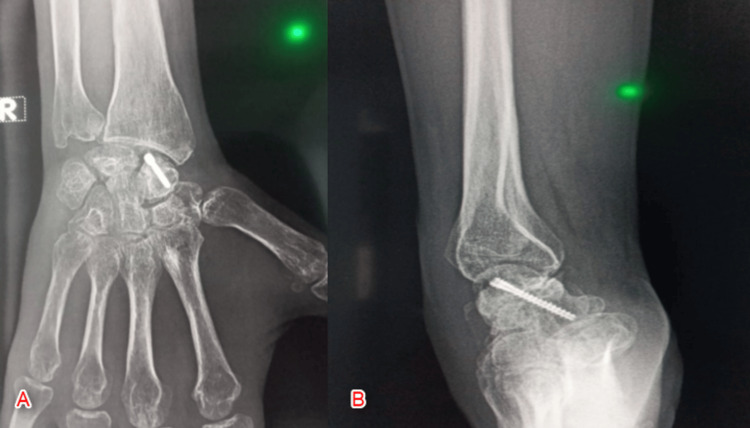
Postoperative X-ray of the right wrist at eight weeks following the removal of K wires and the cast A: Anteroposterior view; B: Lateral view
